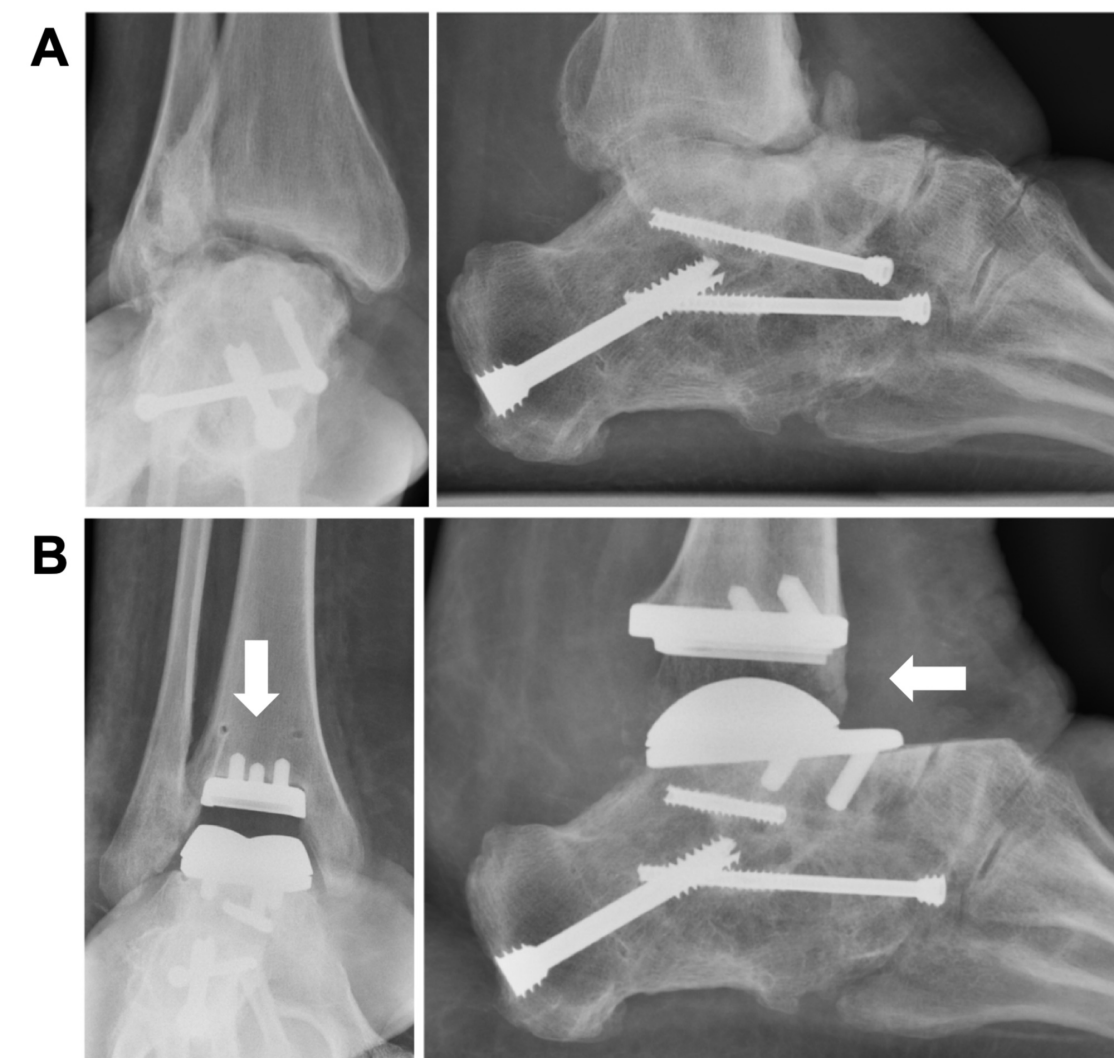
A) Preoperative and B) postoperative anteroposterior and lateral radiographs of an adult patient with previously operated clubfoot with ankle arthritis who underwent total ankle arthroplasty Ankle arthroplasty procedures (arrows) are being performed in adult clubfoot patients but are not yet reported in the literature. Case courtesy of Jeremy McCormick, MD, St. Louis, MO
